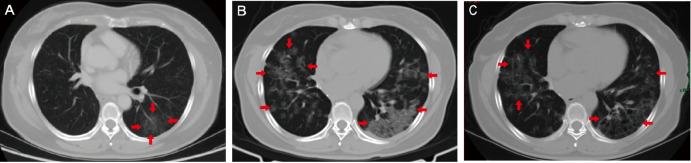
CT scan of lung-mediastinal window after four-cycles of adjuvant chemotherapy (A), at disease progression (B), and achieving lesion aera shrinkage (C)
